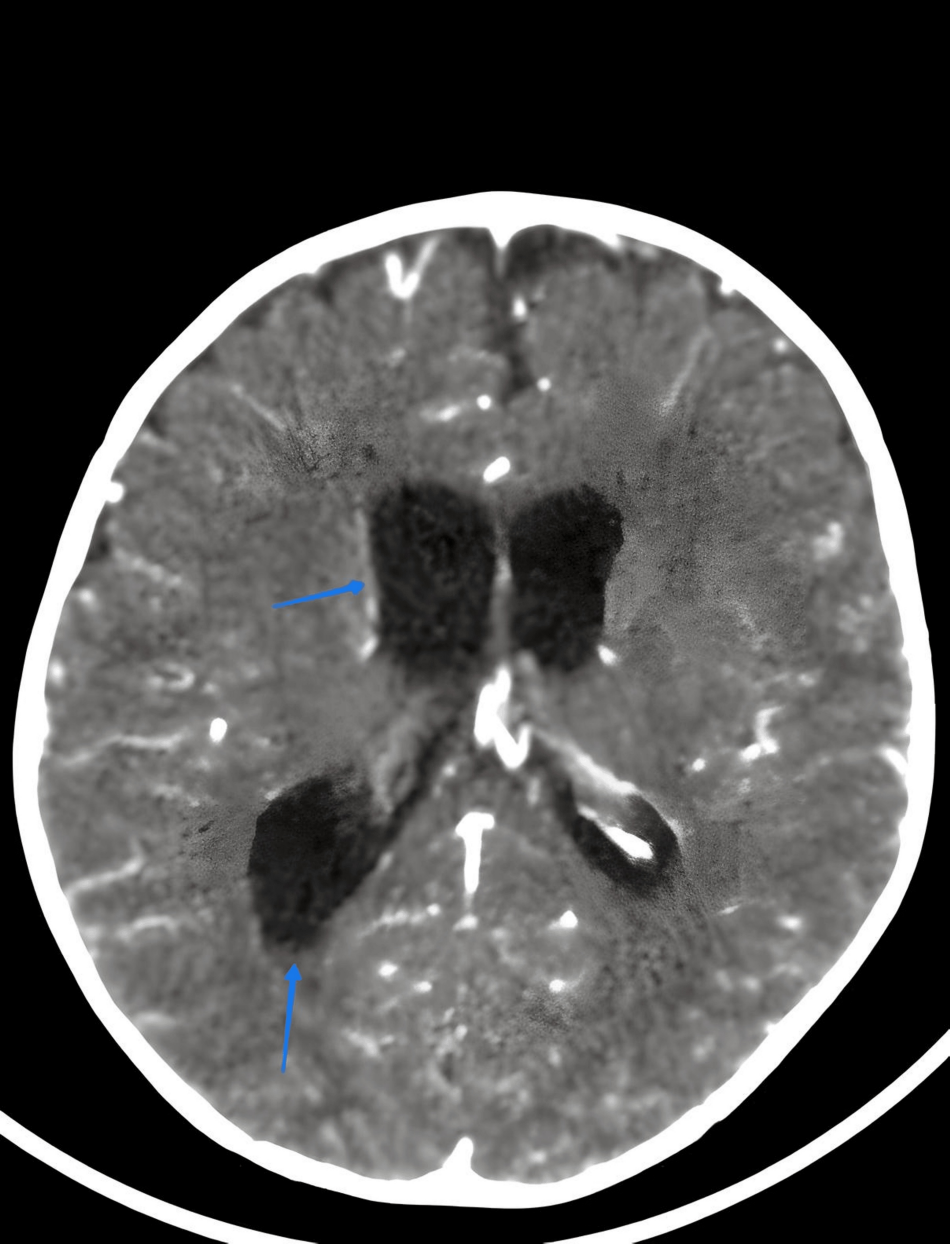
Axial contrast-enhanced brain CT showing triventricular hydrocephalus with right ventricular wall enhancement and occipital horn sediment suggestive of ventriculitis.
